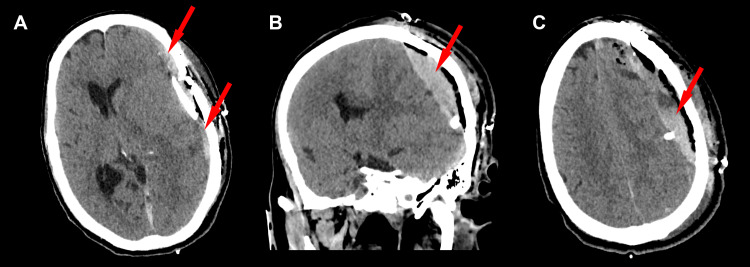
(A) Post-craniotomy day 1 non-contrast CT of the head showing new brain compression and left-to-right midline shift due to acute re-bleeding into the subdural space (red arrows). (B) Coronal view demonstrating a hyperacute left subdural hematoma re-accumulated below the craniotomy site, with diffuse interstitial cerebral edema causing uncal herniation (red arrow). (C) Acute subdural hematoma with associated pneumocephalus (red arrow). CT, computed tomography
